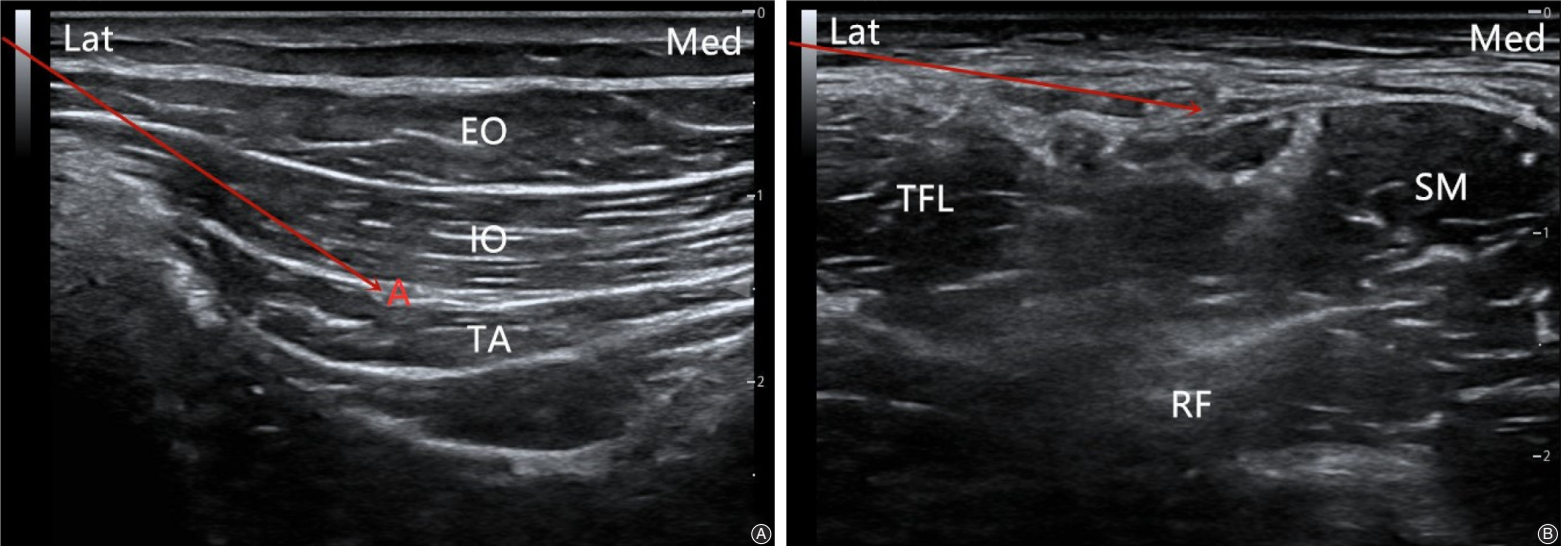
The ultrasound images of IHINB (A) and the lateral femoral cutaneous nerve block (LFCNB) (B). The line shows the needle insertion point. EO, external oblique; IO, internal oblique; RF, rectus femoris; SM, sartorius muscle; TA, transverse abdominis; TFL, tensor fasciae latae.
